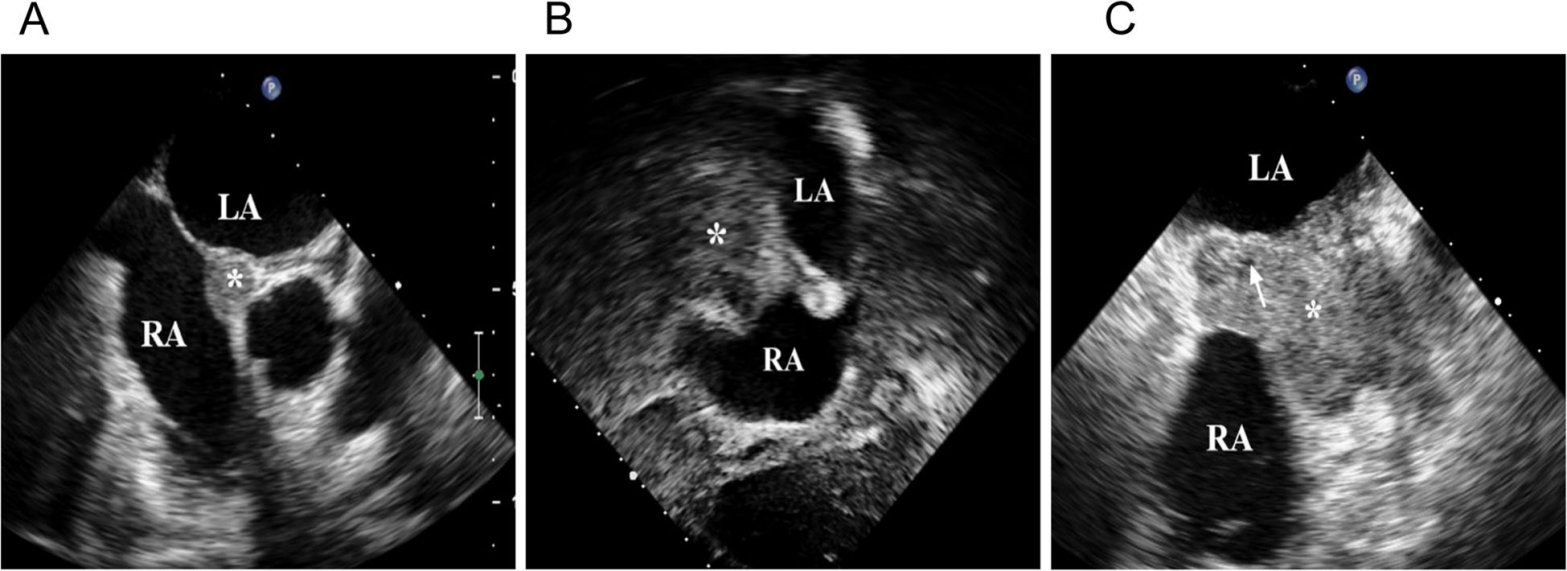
Echocardiography revealed the changes of the mass before and after RFCA. a Before RFCA, transesophageal echocardiography (TEE) revealed a local thickening of the interatrial septum (*) near the root of aorta. b Transthoracic echocardiography (TTE) detected a large immobile, non-pedunculated mass (*) involving the interatrial septum 3 months after RFCA. c TEE revealed a large polymorphic tumor (*) infiltrating the right atrial walls with hypoechoic area (arrow) 3 months after RFCA
